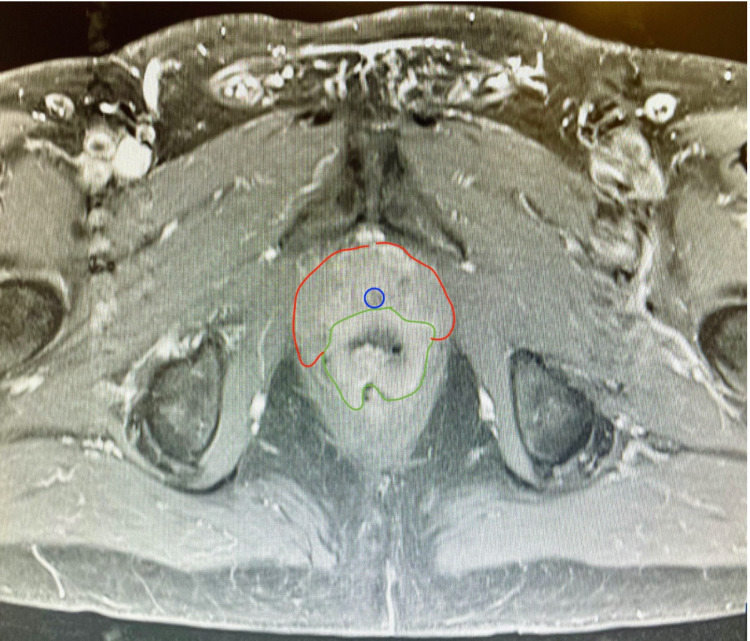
MRI of the pelvis showing the rectal leiomyosarcoma (green) as it invades anterior into the prostate (red) and nears the urethra (blue).
